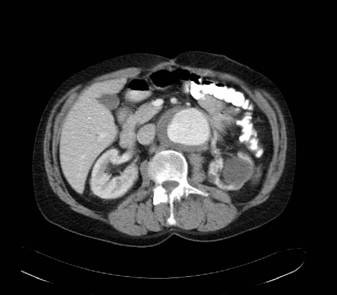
CT scans demonstrating the dissection at the level of the coeliac axis.
